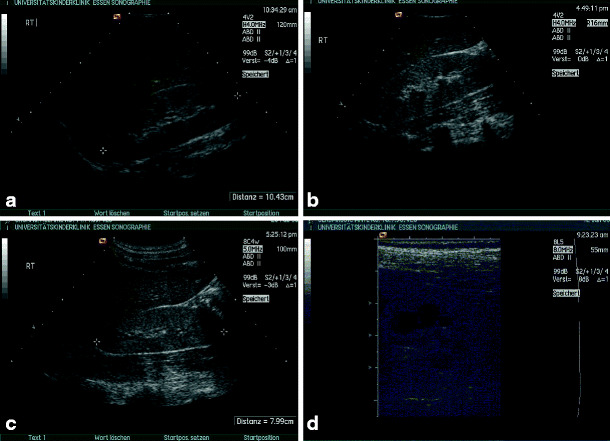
Juvenile nephronophthisis – sonographic appearance: normal or reduced kidney size, enhanced echogenicity of the renal cortex, and reduced corticomedullary differentiation (progressive with renal failure): a 13-year-old boy, creatinine 1.1 mg/dl; b 16-year-old boy (brother of patient in a), creatinine 2.4 mg/dl; c 16-year-old girl, end-stage renal failure; d 9-year-old boy, end-stage renal failure (cysts are a late sign)
